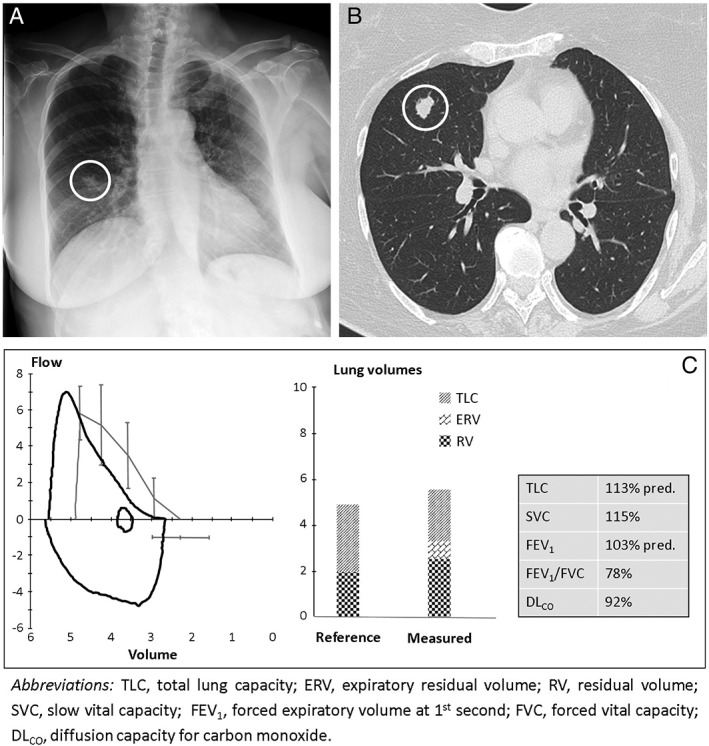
Chest X‐ray and computed tomography (CT) showing pulmonary nodule (A and B, white circles). Lung function testing at baseline (C).
